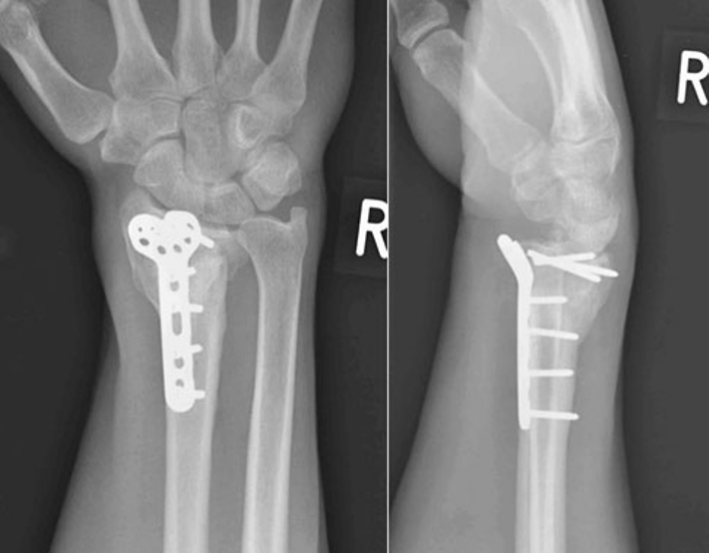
Radiographs at 1 year after second revision
